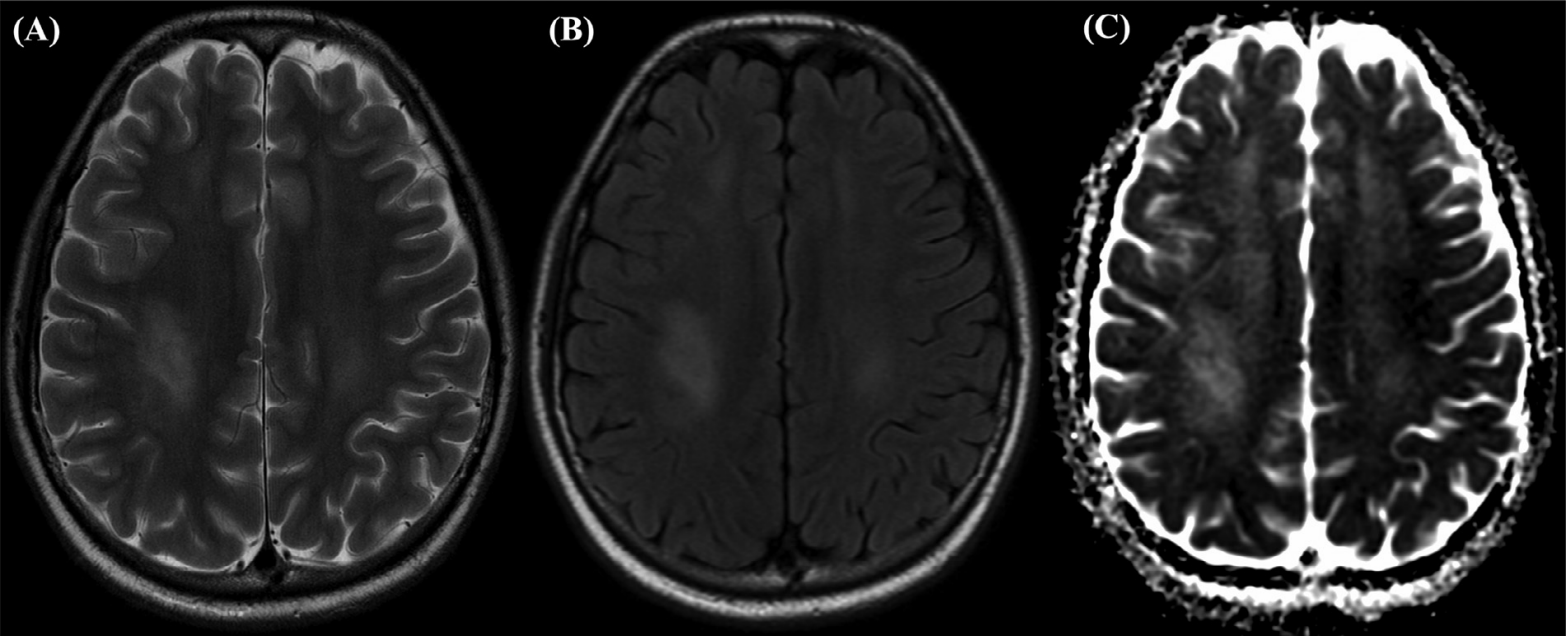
MRI findings taken 20 days after the second episode of MTX-induced LE, revealing further interval increase in the bilateral confluent high T2/FLAIR signal involving the corona radiata and centrum semiovale, however there is no diffusion restriction. These findings are suggestive of chronic leukoencephalopathy on (A) T2-weighted image (B) FLAIR (C) DWI.
